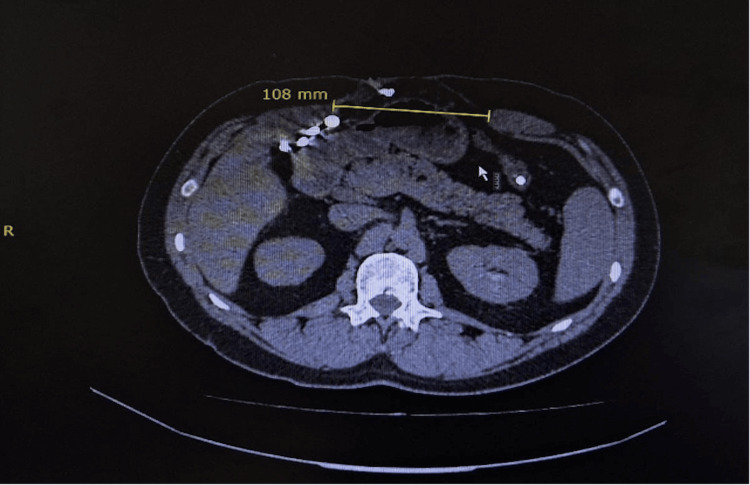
Abdominal CT scan four weeks after botulinum toxin type A administration revealing a reduction in the hernia transverse size to 10.8 cm.
